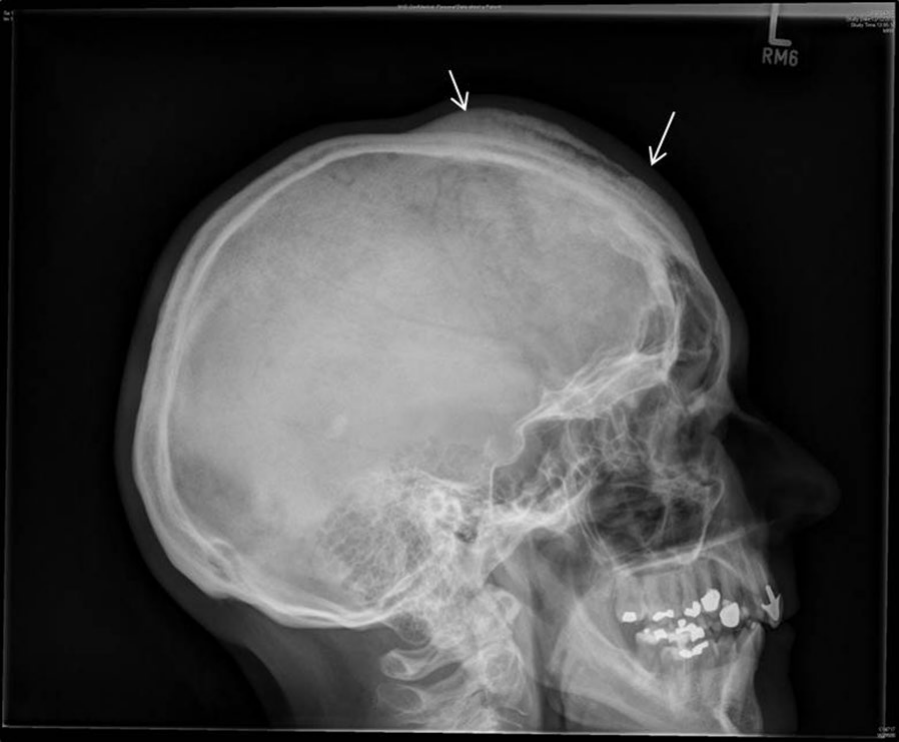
Lateral X-ray of the skull shows an extensive osseous lesion of the diploe with periosteal new bone formation (*arrows*) along the external table of the anterior skull
